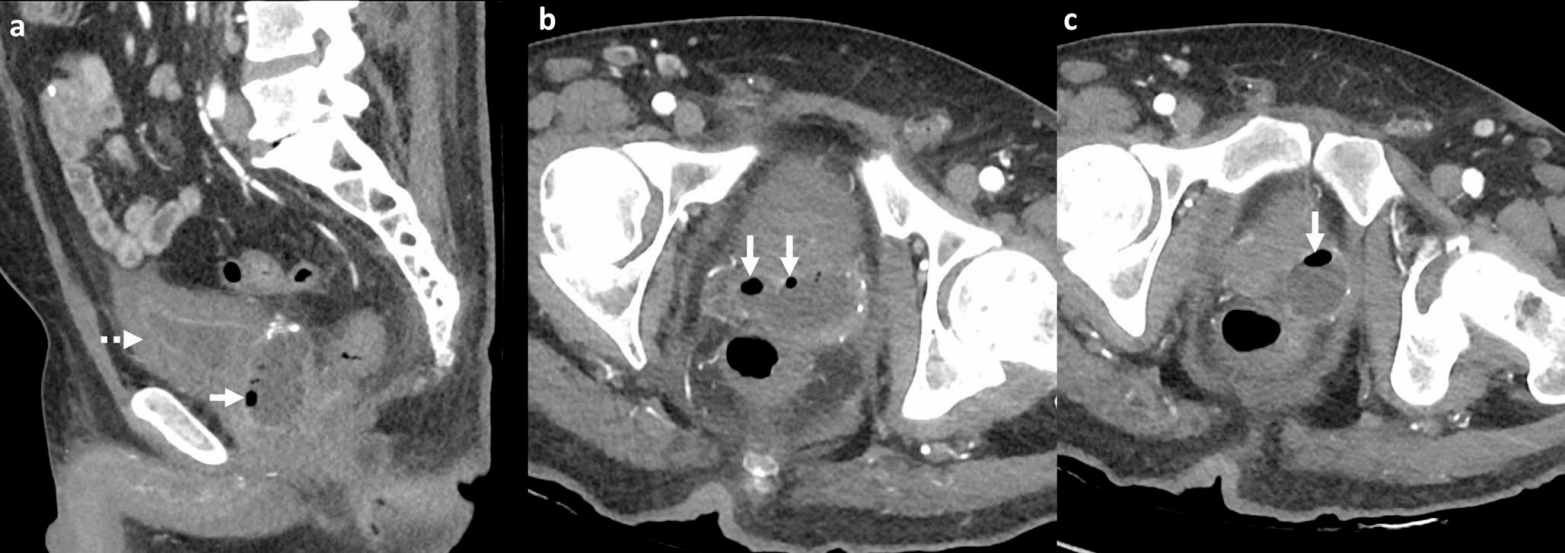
Emphysematous prostate abscess. A 55-year-old with diabetes and end-stage renal disease with fever, dysuria, and bilateral groin pain. CT images show rim-enhancing abscess replacing prostate and bilateral seminal vesicles with air locules (white arrows, a–c) and changes of cystitis (dotted white arrow, a). Blood culture and culture from the abscess showed *Escherichia coli*. The patient was managed with trans-gluteal percutaneous pigtail drain and intravenous antibiotics
